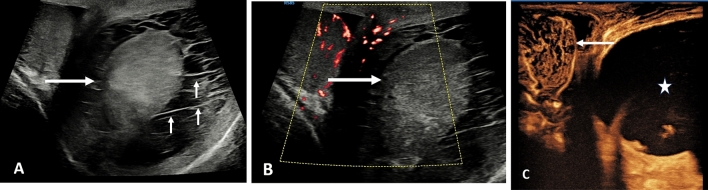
Acute and severe epididymitis and venous infarction of the left testis in a 58 year old. A The B-mode transvers view demonstrates the left testis (arrow) with a septations in a pyocoele (small arrows) in a patient with severe pain and underlying epididymitis. B The microvascular imaging image does not demonstrate any color flow in the left testis (arrow). C On the contrast enhanced ultrasound examination, with a maximum intensity projection, the right testis is adequately perfused (arrow) whilst the left testis (star) has no perfusion following global infarction associated with severe epididymitis
